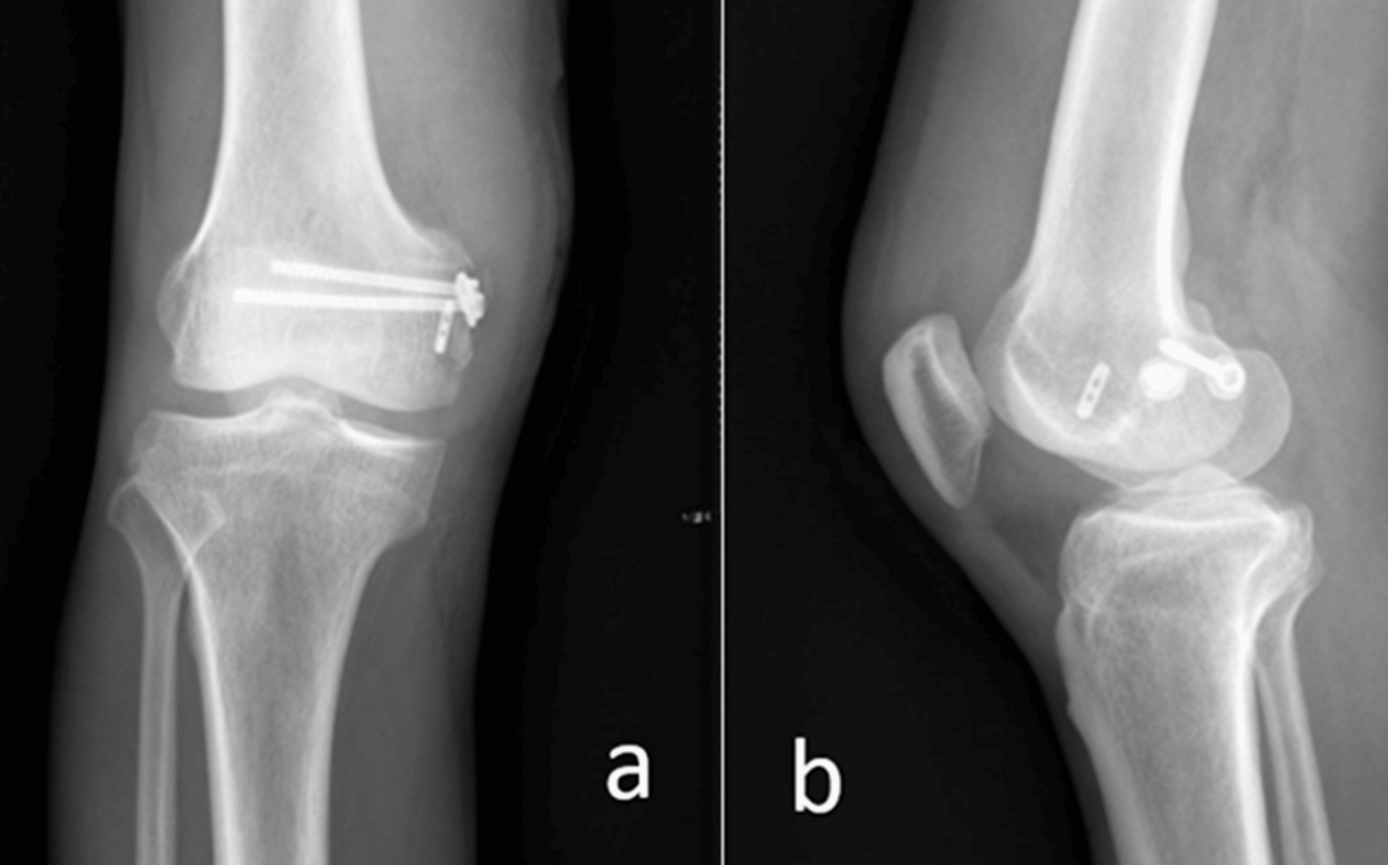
Two-month follow-up X-ray of the right knee. (a) Anteroposterior view and (b) lateral view show satisfactory reduction and healing of the avulsed fragment.
